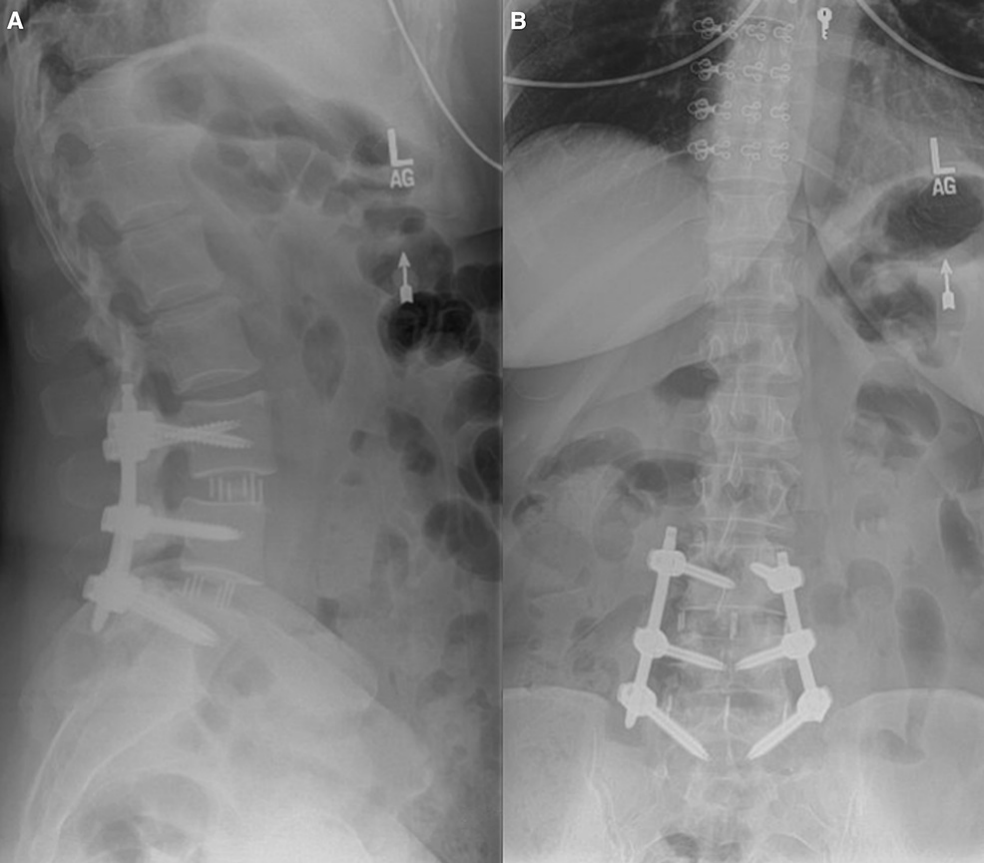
Lateral (A) and AP (B) radiograph of the lumbar spine of the patient at the six-week postoperative period showing improved alignment.
